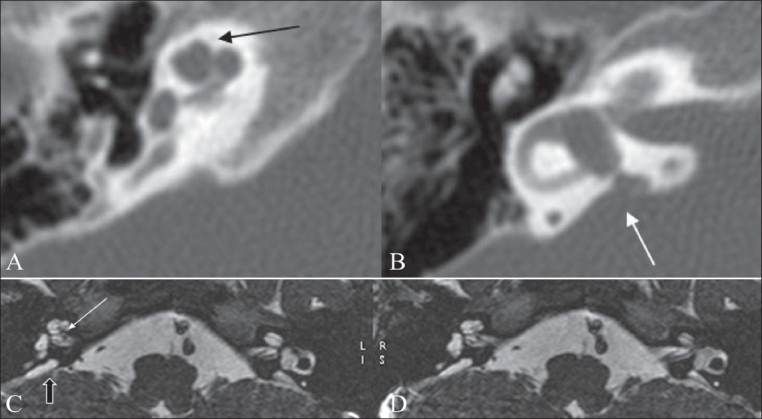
Incomplete partition type II. Axial HRCT images (A, B) show fusion of the middle and apical turns of the cochlea (black arrow in A) and a prominent vestibule and dilated vestibular aqueduct (arrow in B), suggesting an incomplete partition type II (Mondini) deformity. Axial 3D-FIESTA images (C, D) show a dilated vestibular sac (block arrow in C) and a markedly attenuated modiolus (arrow in C)
